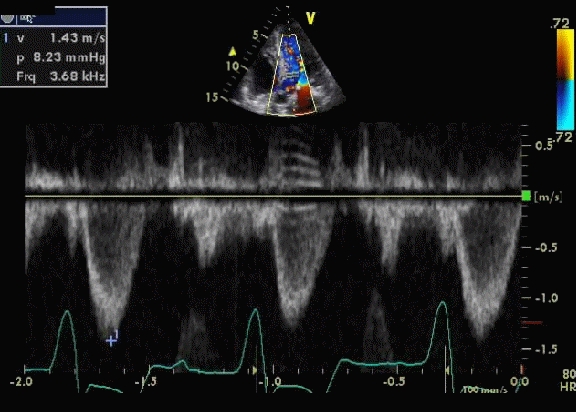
Continuous wave (CW) Doppler image across left ventricular outflow tract during pacing showing a decrease in gradient to 8.2 mmHg ( a decrease of 74 mmHg).
